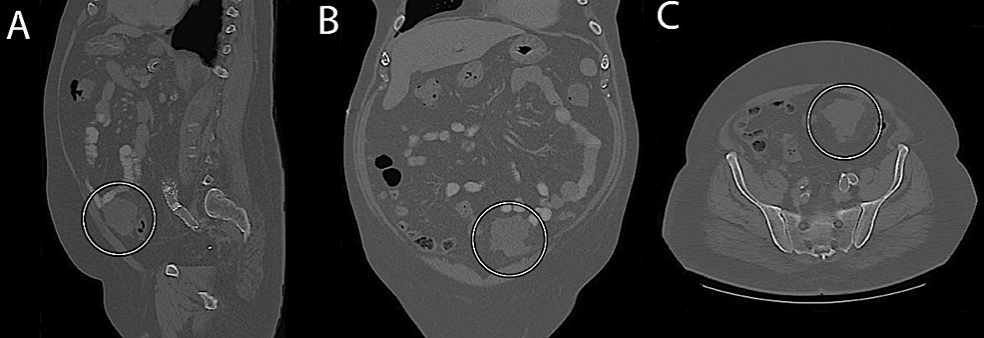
A CT scan prior to surgical resection showed a mesenteric metastatic mass measuring 5.8 cm seen in the anterior lower abdomen (circled in white), along with multiple smaller peritoneal implants throughout the abdomen: (A) sagittal view, (B) coronal view, and (C) axial view.
